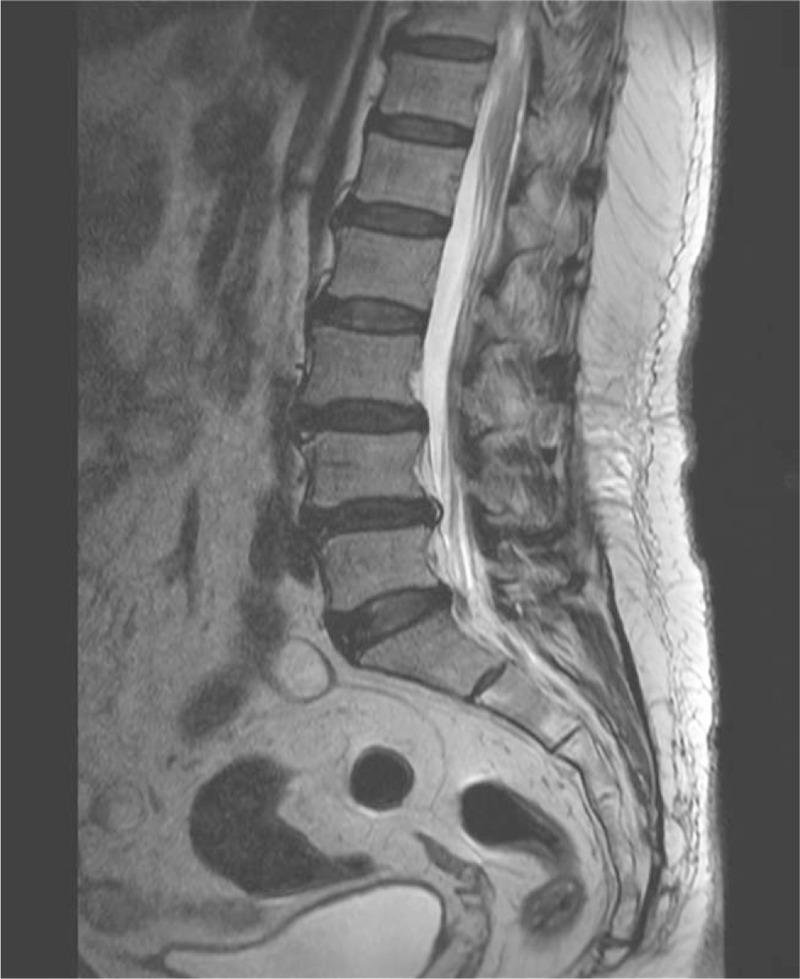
Lumbosacral T2-weighted magnetic resonance image (sagittal view) of L3–L4 and L4–L5 degenerative spondylolisthesis, disk degeneration, and posterior annular fissure at L4–L5.
